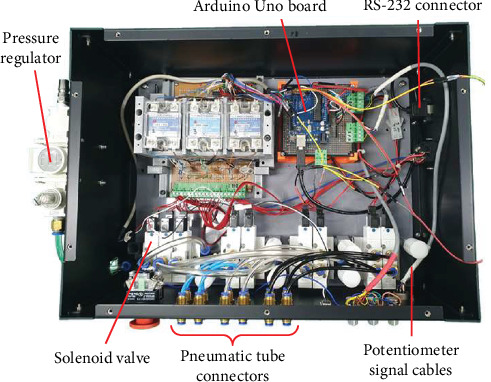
External robotic system controller developed to control transducer movement and HIFU firing at 5 m from MRI bore.
